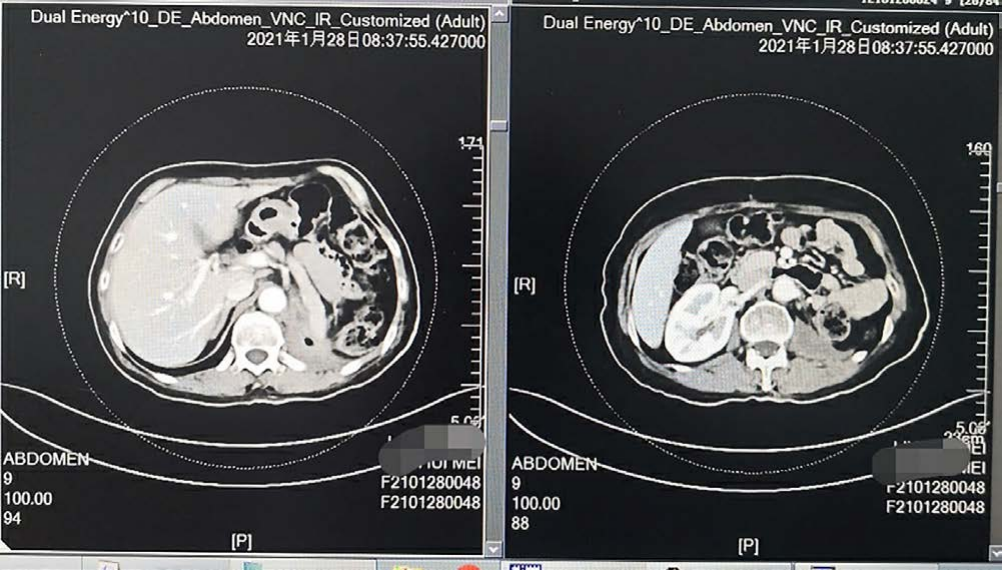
Computed tomography shows the lesions in the abdominal cavity after the regimen of ‘tislelizumab plus apatinib’ was given for four cycles.
